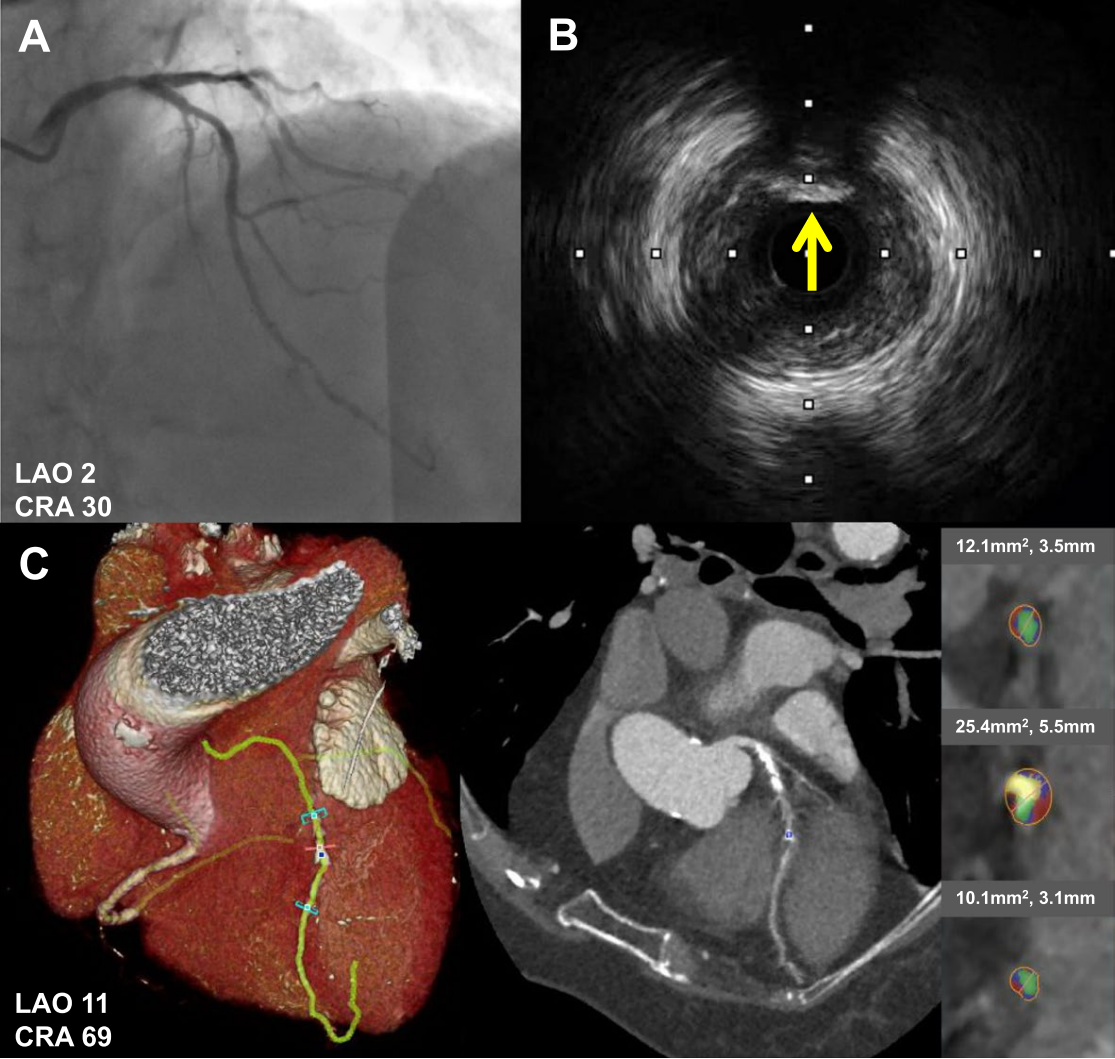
Representative case of quantitative coronary computed tomography angiography (CTA) analysis in patient with calcified nodule. A Invasive angiography showed intermediate coronary stenosis at the proximal to middle left anterior descending. B Intravascular ultrasound confirmed the presence of calcified nodule (yellow arrow). C CTA imaging of plaque with calcified nodule. On the color-coded cross-sectional image, lipid was labeled as red, fiber was labeled as blue and calcium was labeled as yellow
